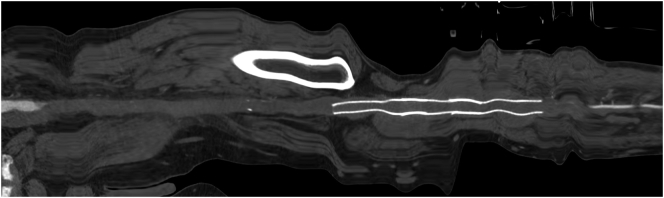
CT angiography with stretched reconstruction showing occlusion of femora-popliteal axis and stent.
